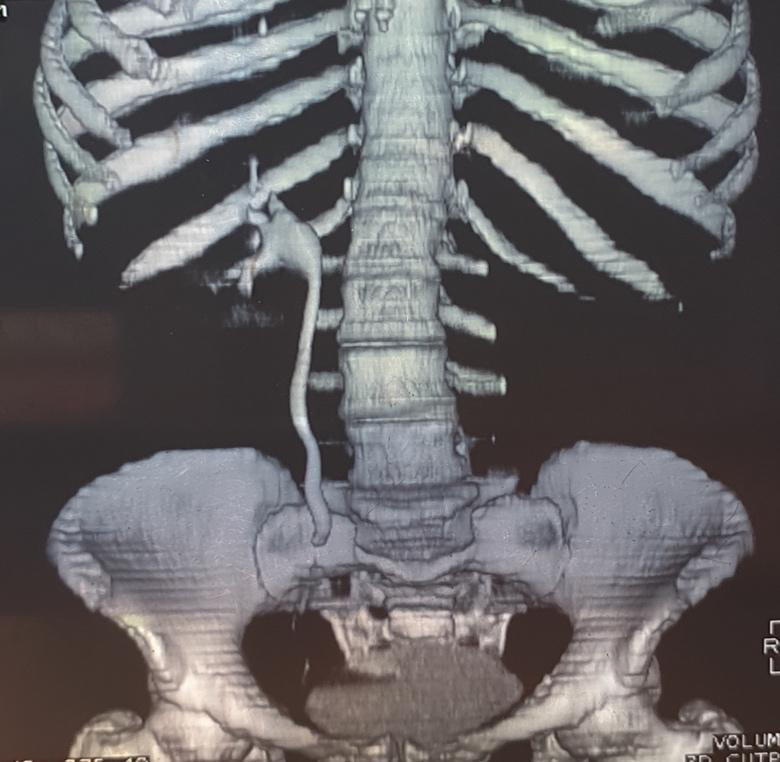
CT abdomen showing normal right pelvicalyceal system and non-visualized left pelvicalyceal system.
